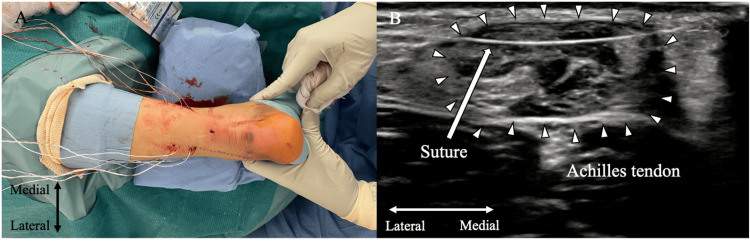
Sutures introduced into the proximal Achilles tendon stump via a needle loop under ultrasound guidance A: External view after suture passage; B: Ultrasound visualization after suture passage Image source: Authors
